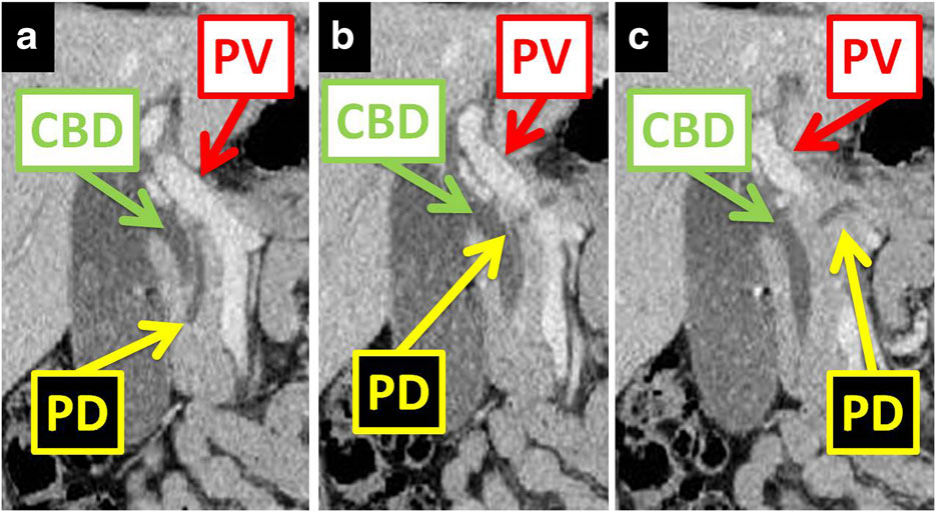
Coronal images of computed tomography of the abdomen. The images show linear configuration of the portal vein and common bile duct, with the pancreatic duct coursing posterior to the portal vein. Three sequential cuts shown: a–c anterior to posterior. *CBD* common bile duct, *PD* pancreatic duct, *PV* portal vein
